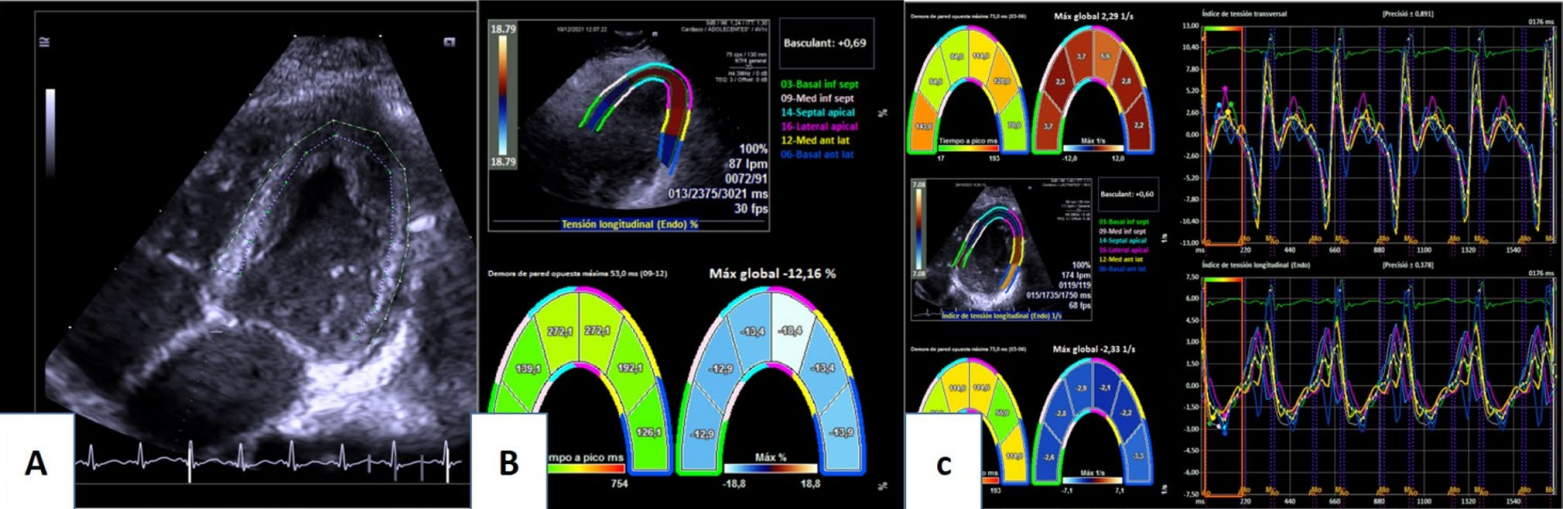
Speckle tracking echocardiography. A Four chambers plane for speckle tracking calculation. B Left ventricular global strain (%). C Strain rate of left ventricle (1/s)
